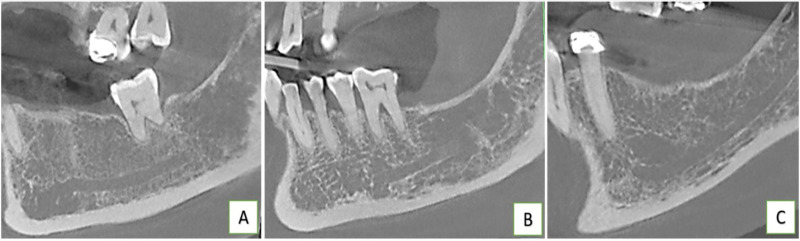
CTCI - computed tomography cortical index types. (A) Type 1 CTCI (B) Type 2 CTCI (C) Type 3 CTCI.
